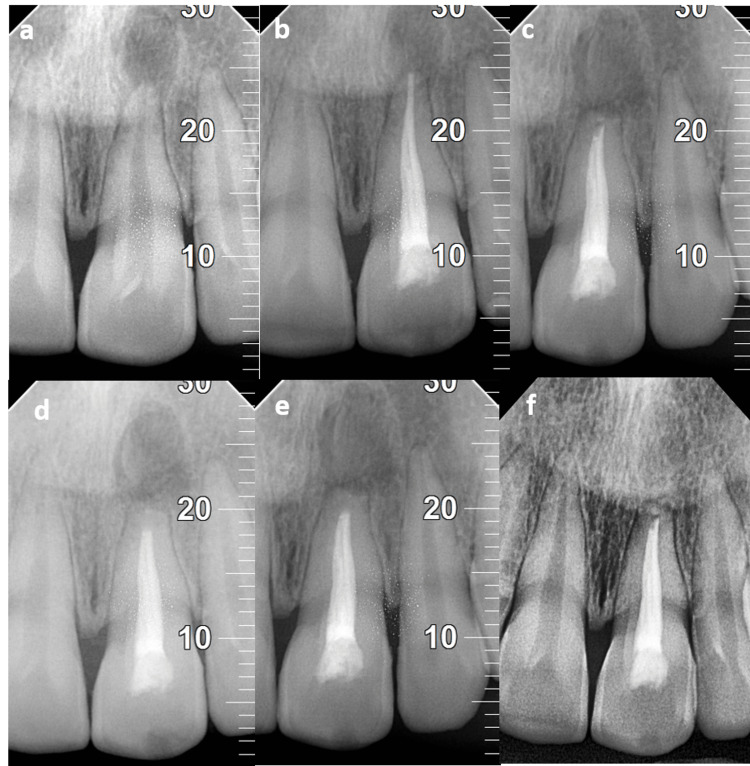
(a) Preoperative radiograph. (b) After root canal treatment. (c) Immediate postoperative radiograph. (d) Three-month follow-up. (e) Six-month follow-up. (f) 12-month follow-up. Markings (10, 20, 30): scale indicating the length and extent of the lesion. It also indicates that the lesion has subsided periodically, as is evident by the measurement.
